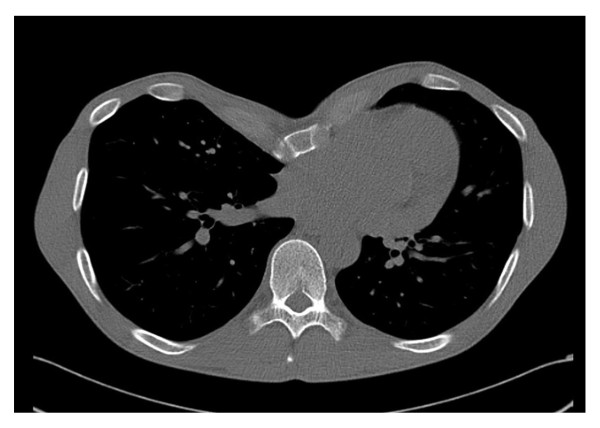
Initial CT-scan showing the pectus excavatum.
